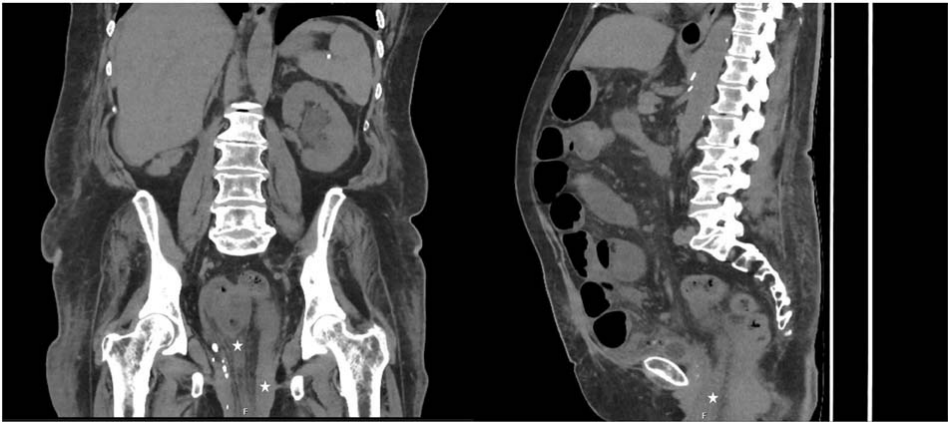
CT coronal (left) and sagittal (right) of abdomen and pelvis, demonstrating extension of small bowel into the pelvis (marked with star) consistent with perineal hernia.
